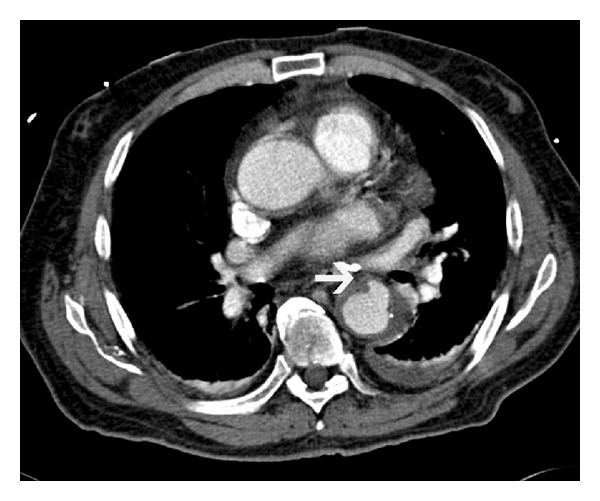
Penetrating atherosclerotic ulcer—axial postcontrast CT scan in a 48-year-old male with acute chest pain shows contrast outpouching from the anterior aspect of descending thoracic aorta (arrow), consistent with a penetrating atherosclerotic ulcer.
